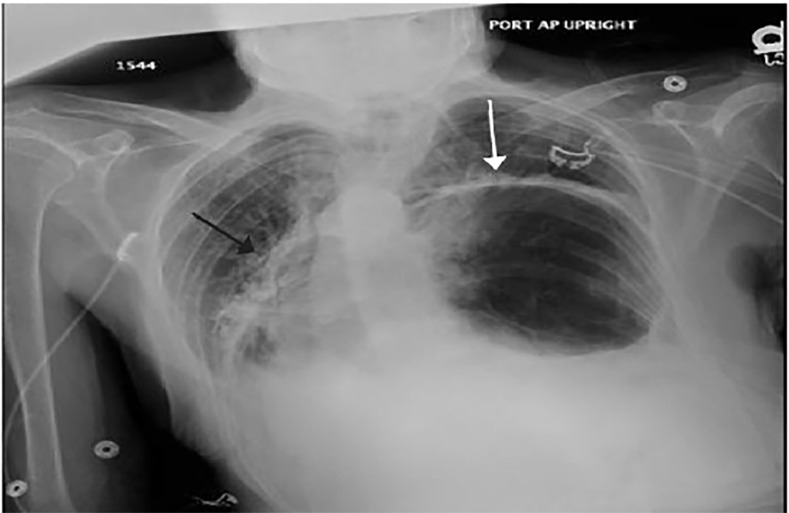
Anterior-posterior chest radiograph demonstrating intrathoracic gastrointestinal contents (white arrow). Note the shift of the mediastinum into the right hemithorax (black arrow).
